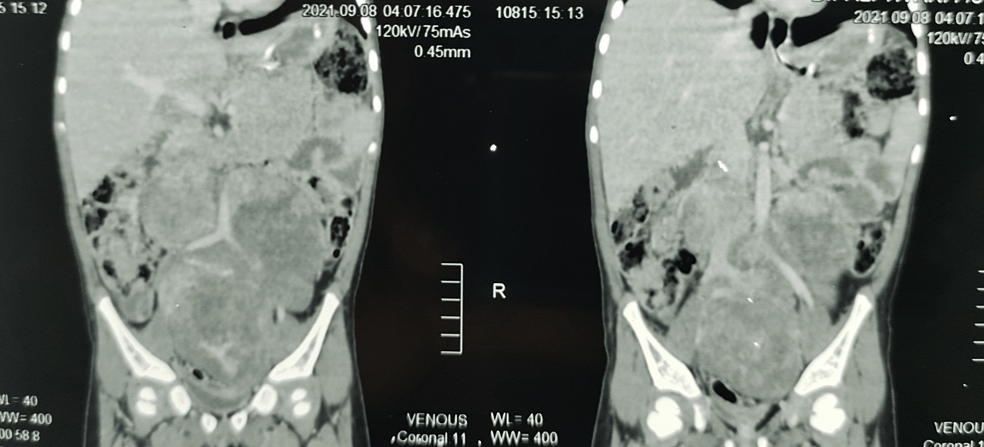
CECT abdomen (coronal section) CECT: Contrast-enhanced computed tomography
